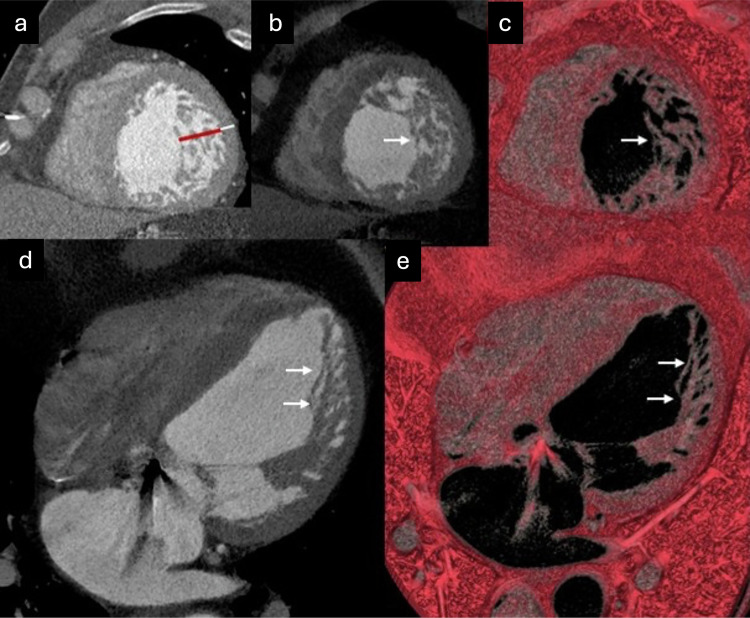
Cardiac CT performed to rule out coronary artery disease in a patient with chest pain incidentally demonstrates LV ET. a Multiplanar reconstruction [MPR] short-axis end-diastolic view showing the trabeculated layer [red line] and compact layer [white line]; (b, d) minimum intensity projection [MinIP] end-diastolic images; and (c, e) volume-rendered [VR] end-diastolic images) show a well-defined trabecular distribution, predominantly located in the lateral wall (arrows)
